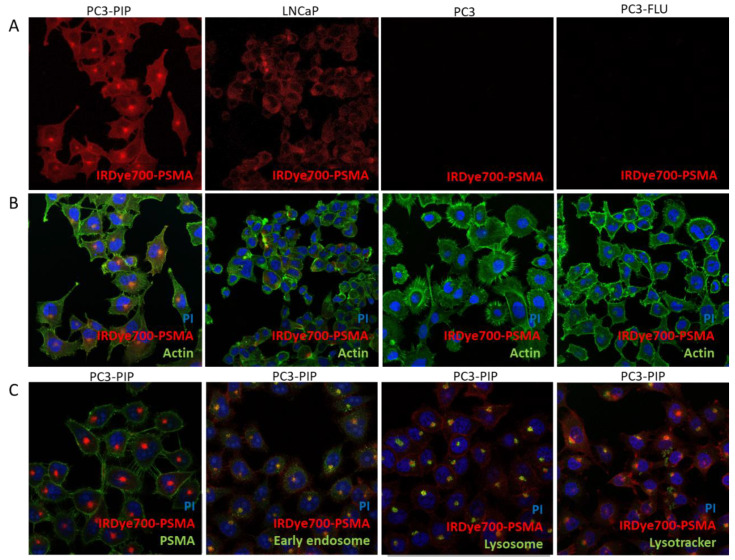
(A) Uptake and cellular distribution in PC3-PIP, LNCaP, PC3 and PC3-FLU of IRDye700DX-PSMA in red; (B) actin in green and nuclei in blue; (C) PC3-PIP cells incubated with IRDye700DX-PSMA in red and nuclei in blue, in green, respectively PSMA, early endosome, lysosome and lysotracker. Magnification 40×, PI 535–617 nm, AlexaFluor488 498–577 nm, IRDye700DX 650–750.
